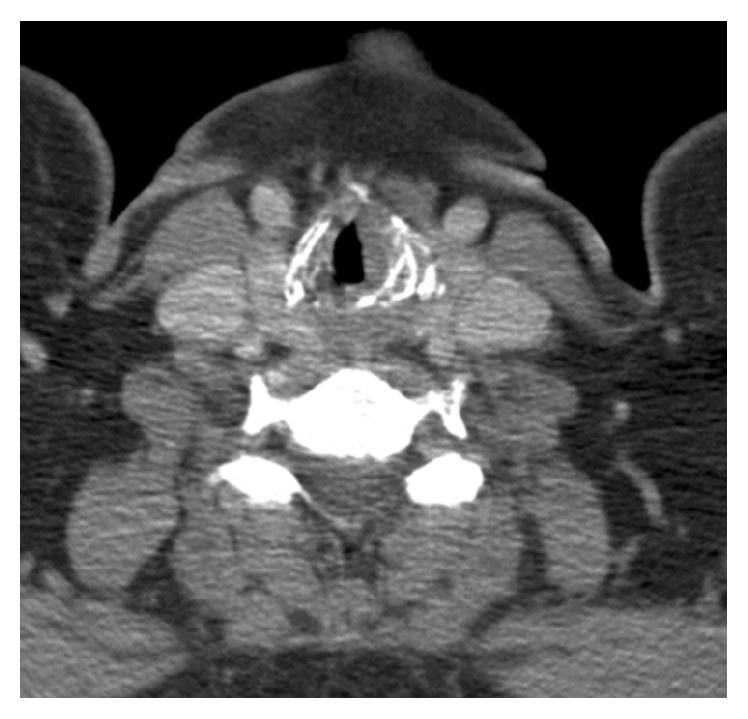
Axial section of CT scan showing left-sided laryngeal asymmetry and homogenous soft tissue mass in the subglottic region.
